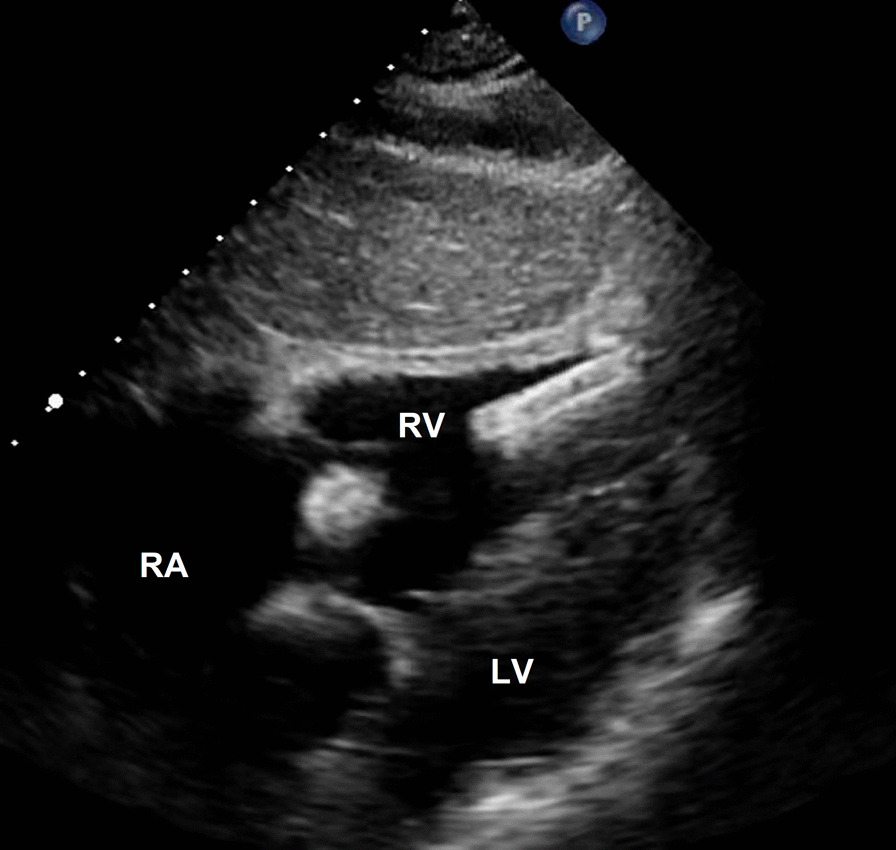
Transthoracic echocardiography shows two hyperechoic tubular shadows at the tricuspid valve orifice and in the right ventricle. LV: left ventricle, RA: right atrium, RV: right ventricle
